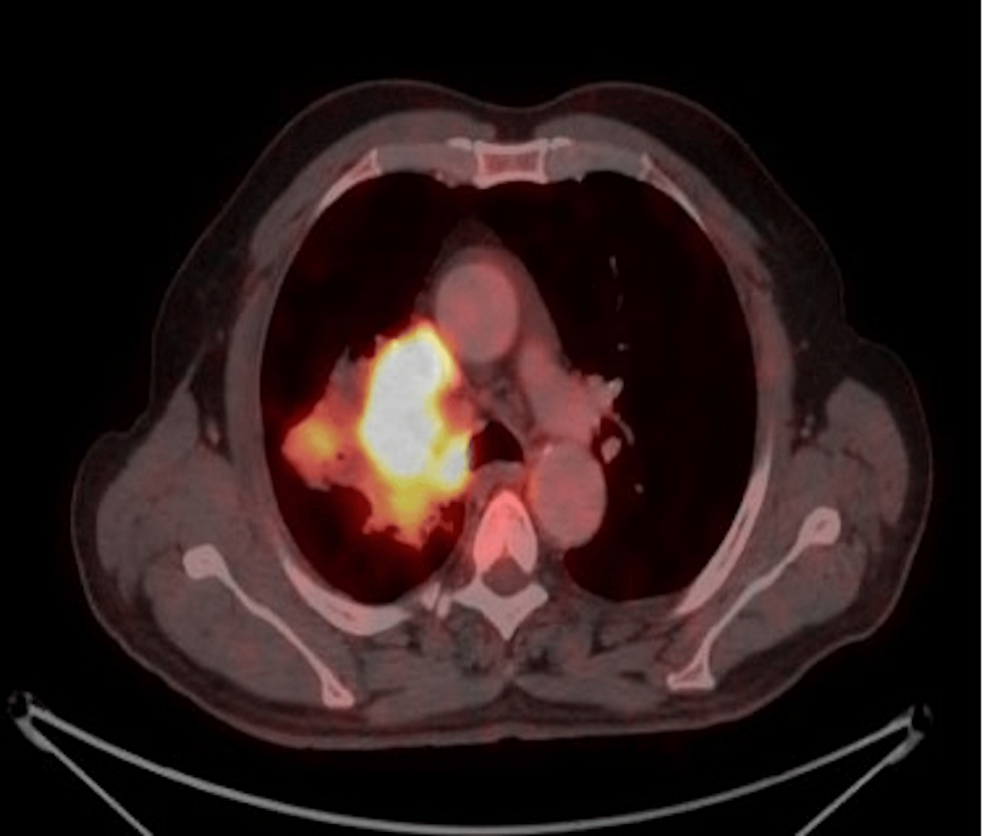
A PET CT image of a 59-year-old patient with a known case of small cell neuroendocrine carcinoma of the right lung. PET-CT was done for initial staging. Intensely FDG avid heterogeneously enhancing irregular lobulated hilar soft tissue mass with areas of necrosis is seen in the right lung, measuring 6.8 x 6.0 x 6.2 cm (AP x T x CC) with SUVmax 23.8.
